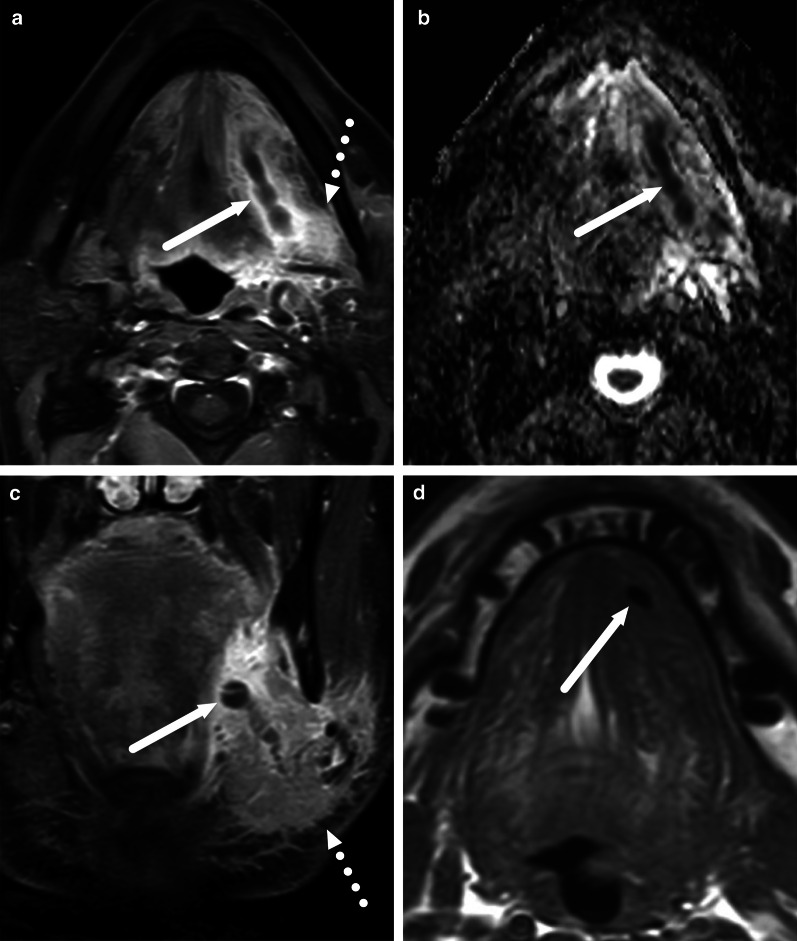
Purulent obstructive sialadenitis of the submandibular gland. Axial (a) and coronal (c) post-contrast fat-suppressed T1-weighted images show a dilated submandibular duct (Wharton’s duct) (arrow), and significant edema in and around the gland (dotted arrow). ADC map confirms that the dilated duct is filled with pus (arrow) (b), and axial T1-weighted imaging at a level slightly superior shows a sialolith as an ovoid area of signal void (arrow) (d)
